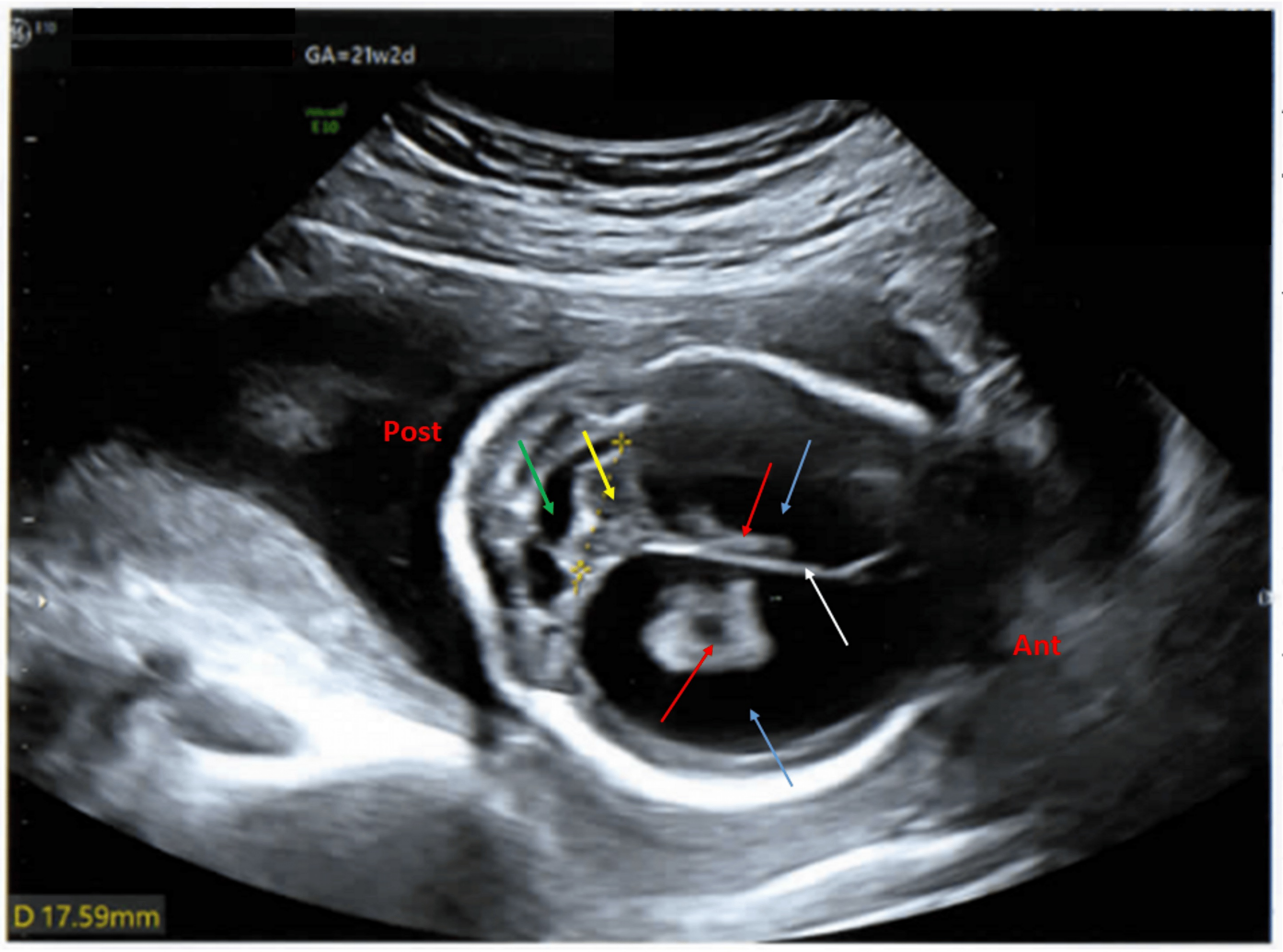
Antenatal ultrasound of the fetus in the second pregnancy at around 21 weeks 2 days gestation showing cerebral ventriculomegaly and cerebellar hypoplasia Antenatal ultrasound of the fetus in the second pregnancy showed a transverse view of the fetal skull at the level of the posterior fossa (ant: anterior, post: posterior), around 21 weeks 2 days gestation, showing dilated cerebral ventricles (blue arrows), cerebellar hypoplasia (yellow arrow), the trans-cerebellar diameter was 17.59 mm (6 percentile, z score is minus 1.59), cisterna magna was normal (green arrow), and falx cerebri was normal (white arrow).
